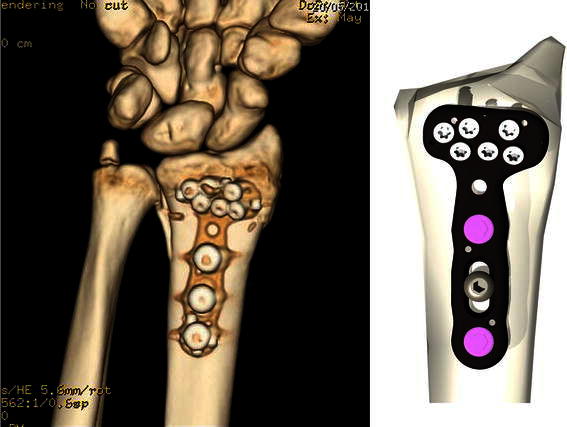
3-D reconstruction of the new DiPHOS-RM plate and CT scan in 3-D showing an implanted plate in a C1-type fracture
